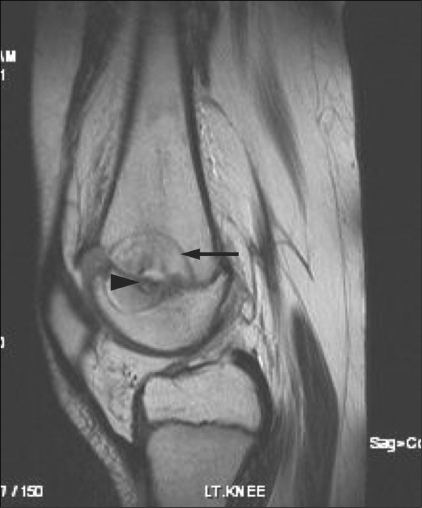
Follow-up MRI after RFA of a subphyseal femoral osteoid osteoma of the femur. A sagittal T1W MRI (A) shows an area of coagulation necrosis (arrow) surrounding the nidus (arrowhead). A coronal contrast-enhanced T1W MRI with fat suppression (B) shows a hypointense perfusion defect (arrow) surrounded by a thin enhancing rim (arrowhead)
